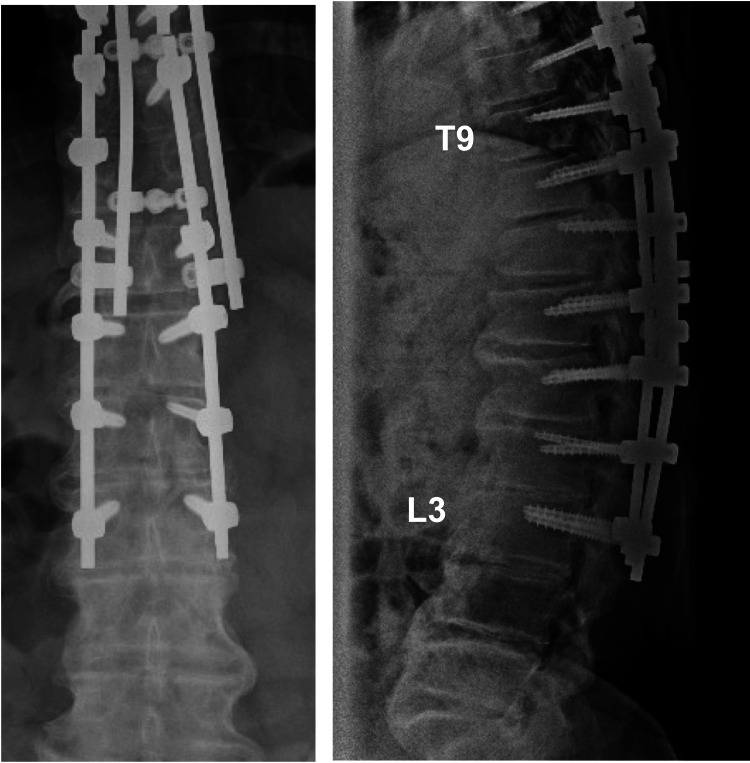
Radiographs after revision surgery Radiographs after removing the recurring OLF at the T10/11 and extending the posterior fixation to the L3. OLF: ossification of the ligamentum flavum
